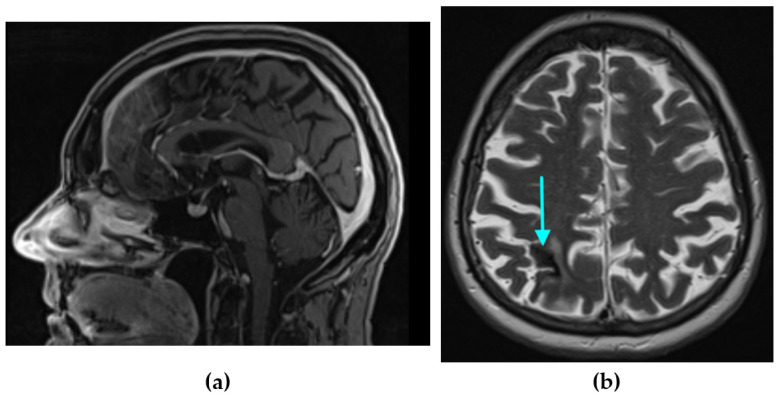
Follow-up brain MRI at 90 days demonstrates (a) post-contrast sagittal 3D T1-weighted MPRAGE sequence showing recanalization of the superior sagittal sinus and (b) axial T2-weighted TSE image showing interval reduction in the right parietal hemorrhage (arrow).
